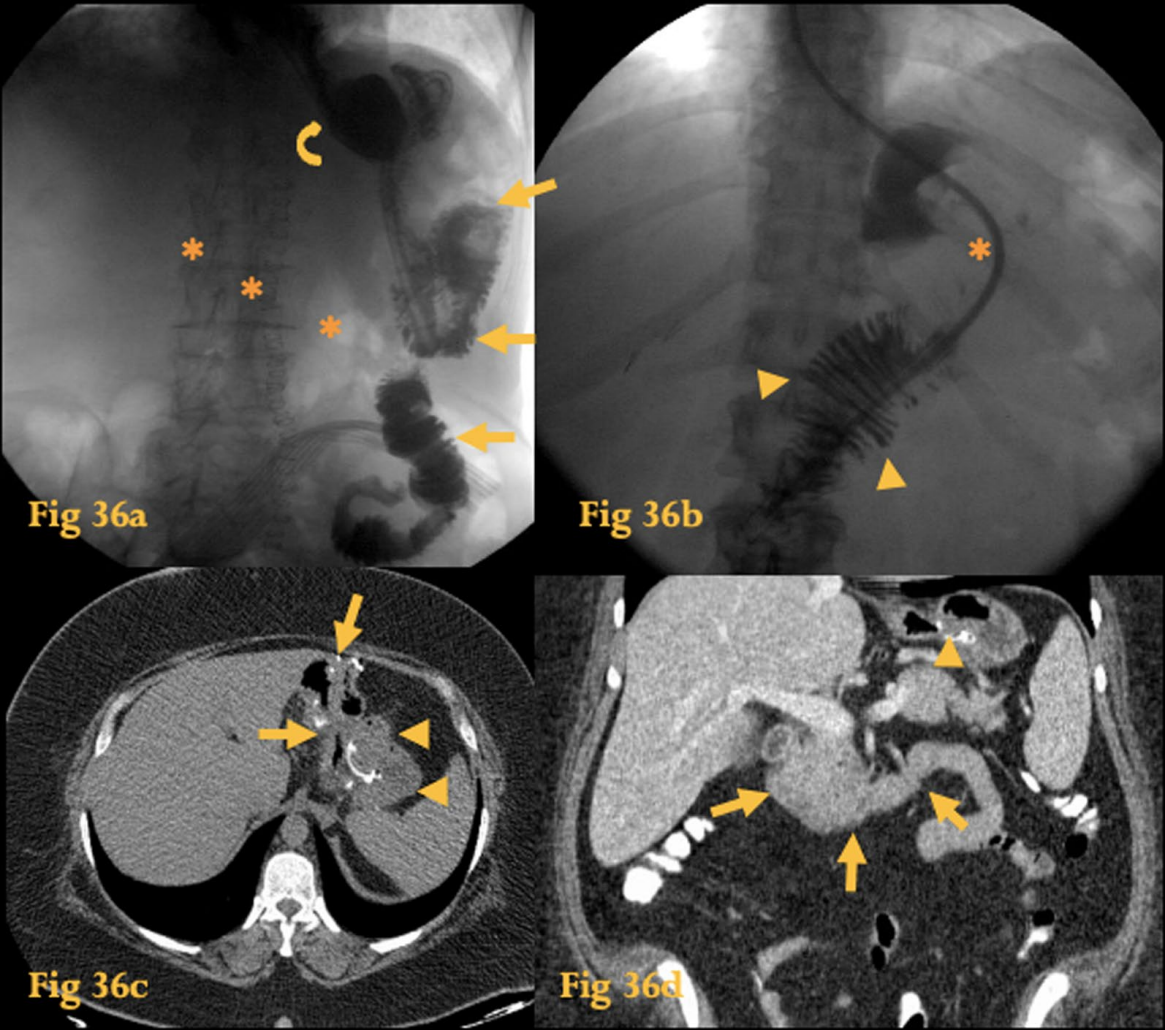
Roux-en-Y anastomosis. UGIS with water soluble contrast of a patient who underwent partial gastrectomy with Roux-en-Y anastomosis for an adenocarcinoma of the stomach (a): only the gastric stump (curved arrow) and gastro-jejunal loop are opacified (arrows); the biliopancreatic loop is not (asterisks in its supposed location). Fluoroscopic study with water soluble contrast of an obese woman who underwent gastric bypass with Roux-en-Y anastomosis (b): note the jejunal mucosal pattern (arrowheads) right after the supposed location of the gastro-jejunal anastomosis (asterisk). Abdominal CT of the same patient (c): the surgical clips are seen at the anastomosis between the gastric stump (arrows) and the jejunal loop (arrowheads). CT coronal reformation (d) shows the gastro-jejunal anastomosis (arrowhead) and the biliopancreatic limb, which includes the duodenum in its normal location (arrows)
